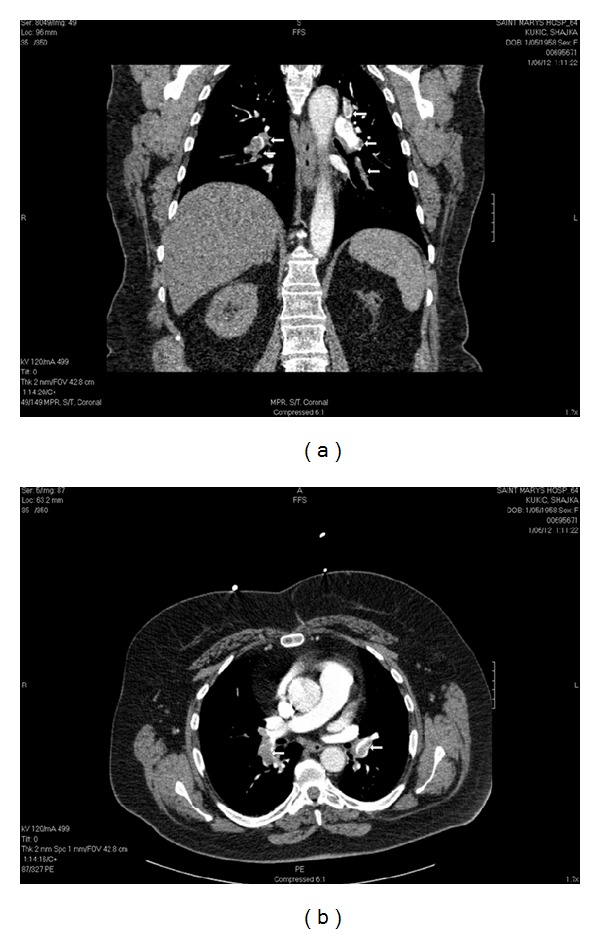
CT scan of chest, coronal view. White arrows point to bilateral pulmonary emboli.
